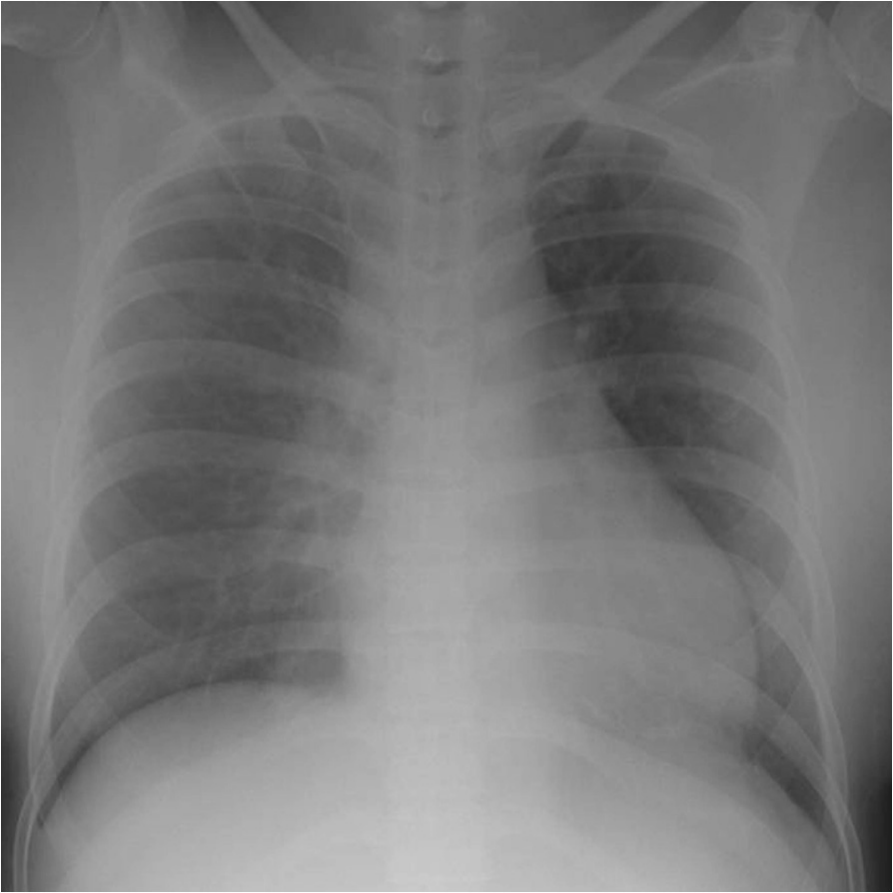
Chest radiography on admission. Taken in the semi-sitting position. Shows cardiomegaly (cardiothoracic ratio, 55%), reduced radiolucency of both lung fields, and prominent pulmonary vascular markings.
